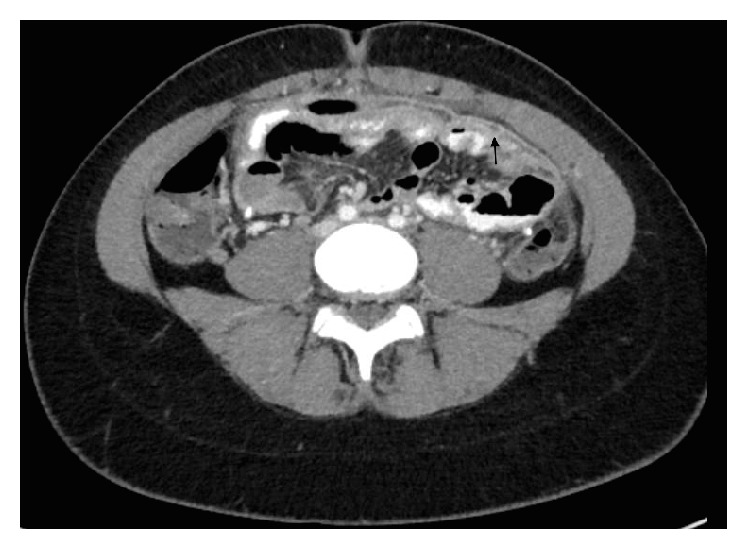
Peritonitis due to NVS. Abdominal CT scan with contrast. The arrow points to the hyperintensity demonstrating fluid accumulation consistent with peritonitis.
